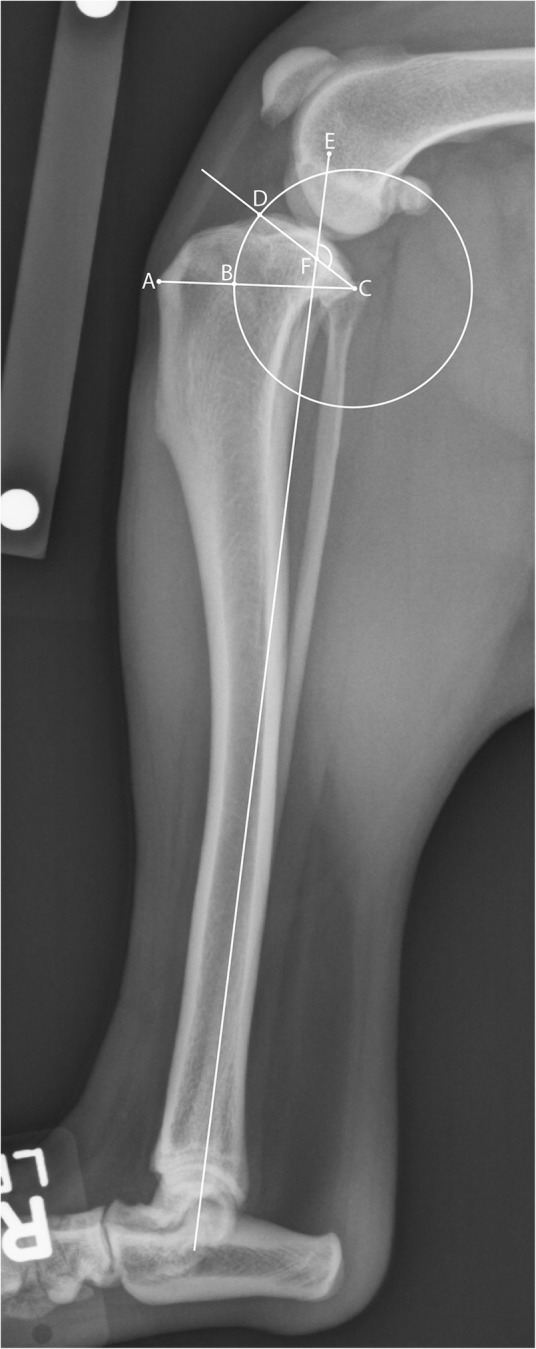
Measurements of proximal tibial morphology taken from a lateral stifle radiograph. Before measurement, the radiograph was evaluated for appropriate technique and position. A. The most anterior point of the tibial tuberosity. B. The cross point of a circle on line AC where the center is at the most posterior point of the tibial plateau (C) and also crosses the most anterior point of the tibial plateau (D). C. The most posterior point of the tibial plateau. D. The most anterior point of the tibial plateau. E. A reference line extending from the center of the intercondylar eminence proximally to the center of the tarsus distally. F. The cross point of line E and the tibial slope line (DC). Tibial plateau angle (TPA) was measured as the obtuse angle at EFC - 90°. Relative tibial tuberosity width (rTTW) was measured as the width of the tibial tuberosity divided by the width of the proximal tibia not including the tibial tuberosity (AB/BC)
